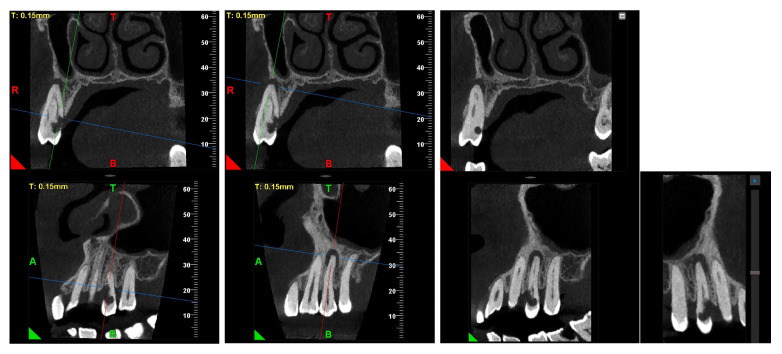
Pano-rex and cross-sectional CBCT images of tooth 1.4, showing its ECR, EPL, and PL. The colored lines crossing the images represent the orthogonal planes (axial, coronal, and sagittal) in the multiplanar reconstruction. Their correct use and orientation allow for an accurate identification of the extent of the lesions, including possible relationships with the maxillary sinus, in all spatial planes and a detailed analysis of the endodontic anatomy. The green and red letters are the coordinates of the image: A: anterior, R: right, B: bottom, T: Top.
